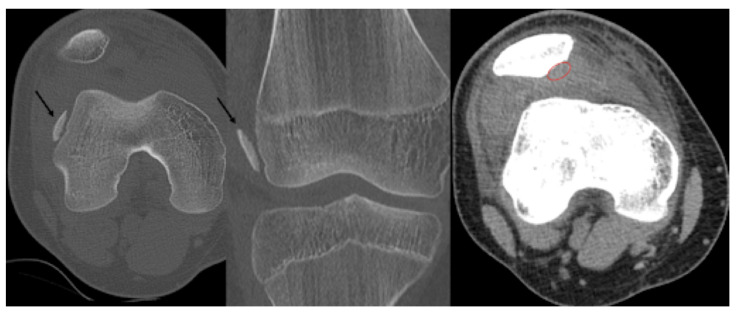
CT images of the knee, showing the fragment (black arrow) and the location of the missing piece (red circle).
